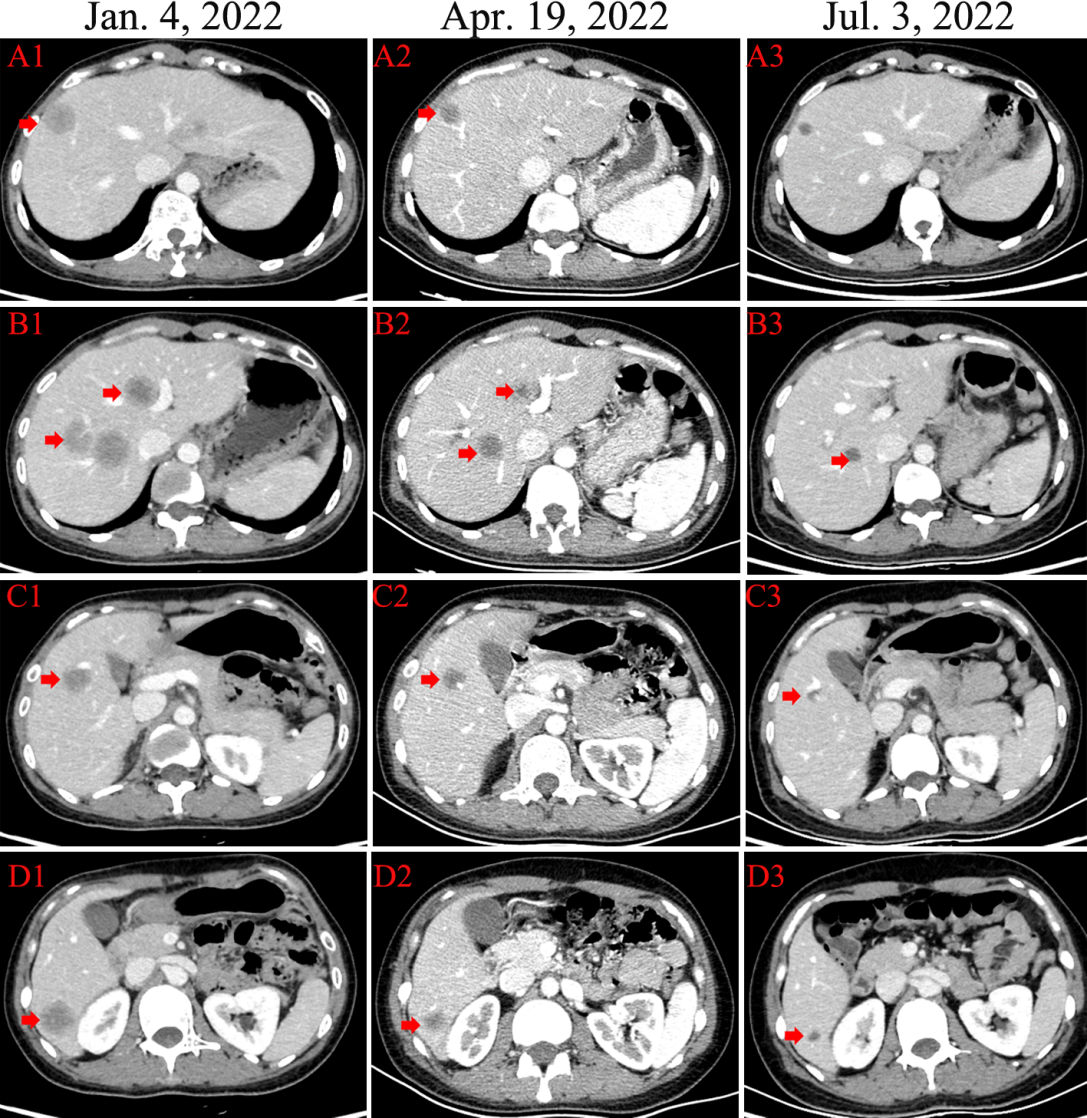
The CT changes of different metastatic lesions from liver (A–D).
